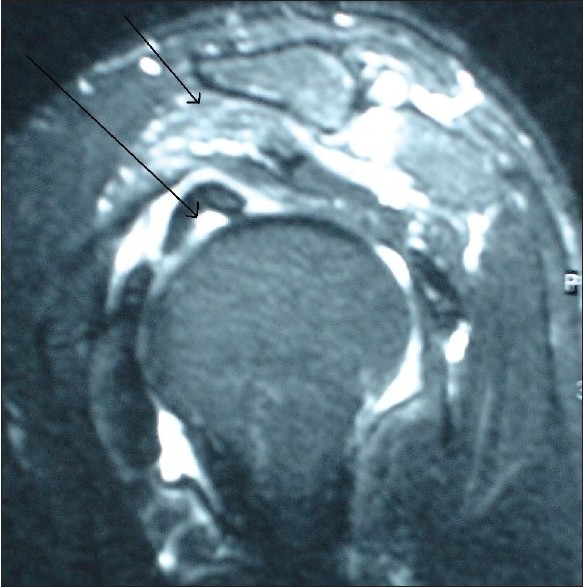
Shoulder MRI. The left arrow demonstartes the intra-articular LHB stump. The right arrow is pointing to the os acromiale
